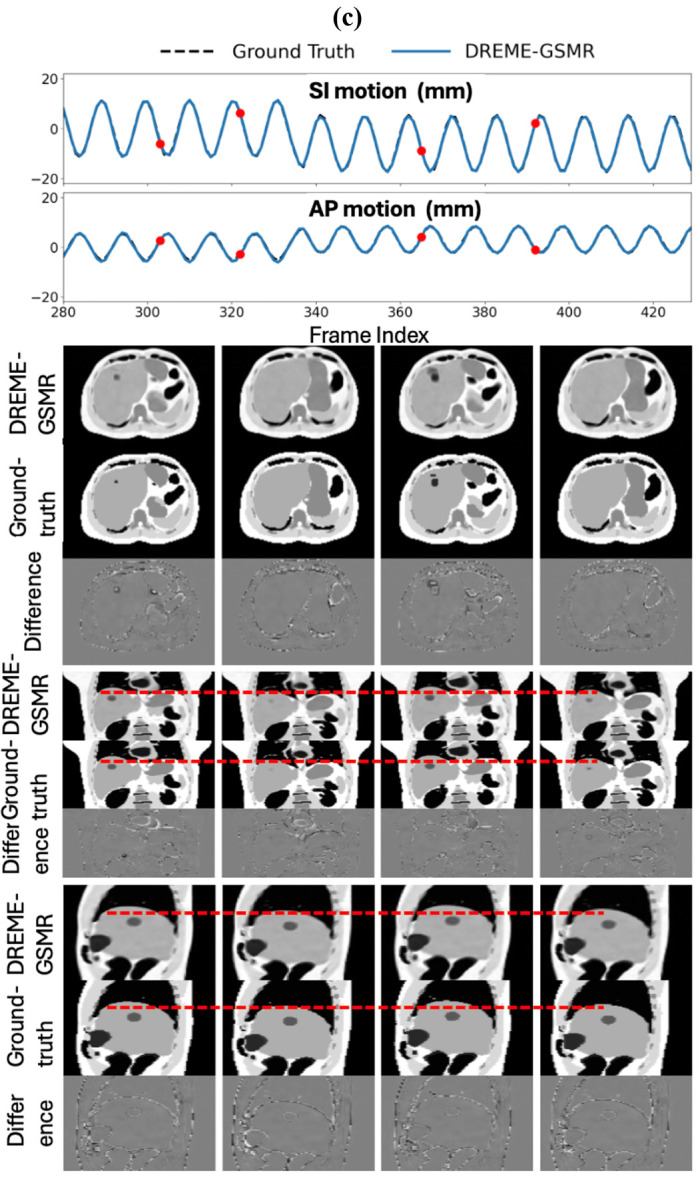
XCAT study results visualizations. (a) Comparison of reconstructed reference-frame MRIs from six motion scenarios (X1-X6, from left to right) between DREME-MR and DREME-GSMR. (b) Liver tumor center-of-mass trajectories in the SI direction of the XCAT study. The DREME-MR and DREME-GSMR were trained on the X1 scenario and tested on all six (X1-X6) scenarios. (c) An example of DREME-GSMR’s dynamic reconstruction results in the XCAT study (training case X2). The first two rows show the estimated motion curves along the SI and AP directions. The following rows compare the reconstructed MR images with the ‘ground-truth’ images at four time points at axial, coronal, and sagittal views. The window widths of the difference images are half of those of the MR images.
